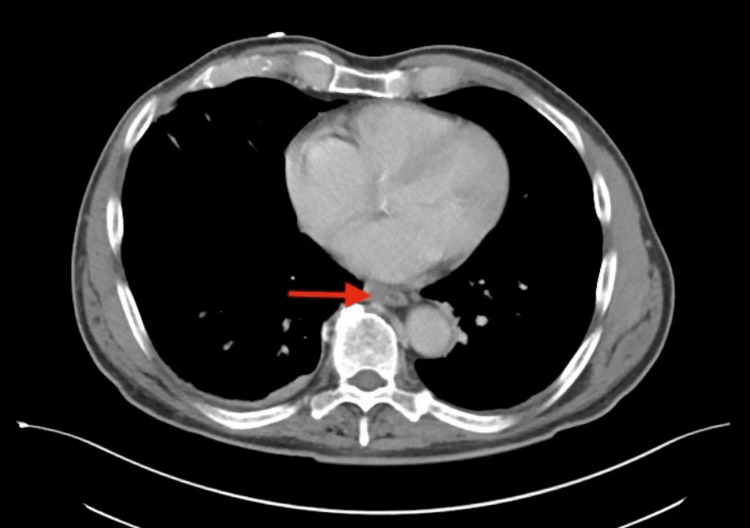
Axial contrast-enhanced computed tomography of the chest The image demonstrates circumferential thickening of the mid-to-distal esophagus (arrow) without focal mass or obstruction. The significance of this finding is uncertain and may represent a nonspecific inflammatory response.
